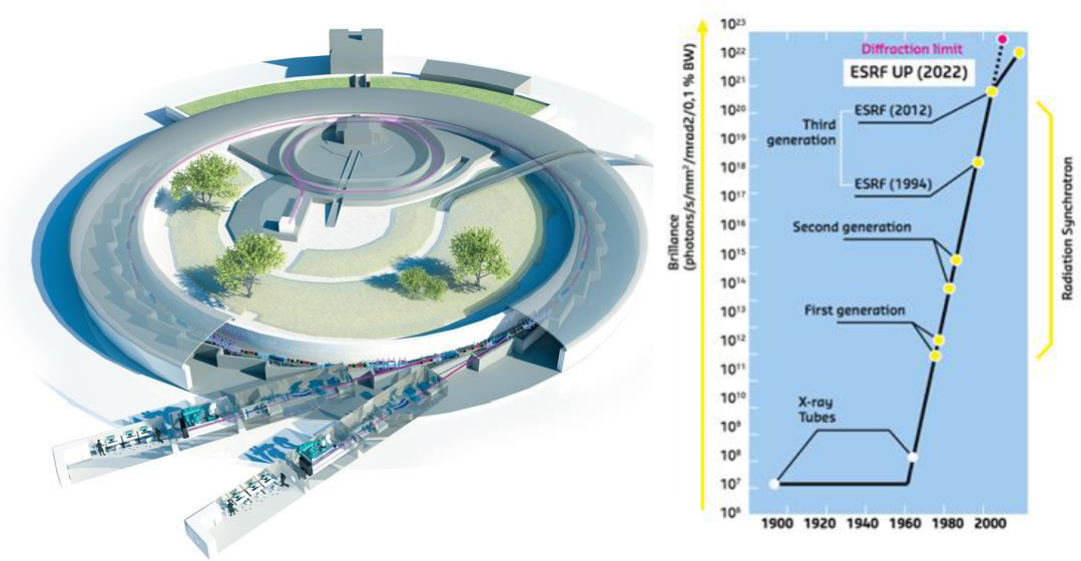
A representation of a synchrotron storage ring, including linear accelerator, booster and two beamlines (left) and the increase in X-ray brilliance since the first X-ray tubes to the current ESRF configuration and the predicted next generation after the machine upgrade planned in 2020. Credit: S. Gerlier/ESRF with permission.
